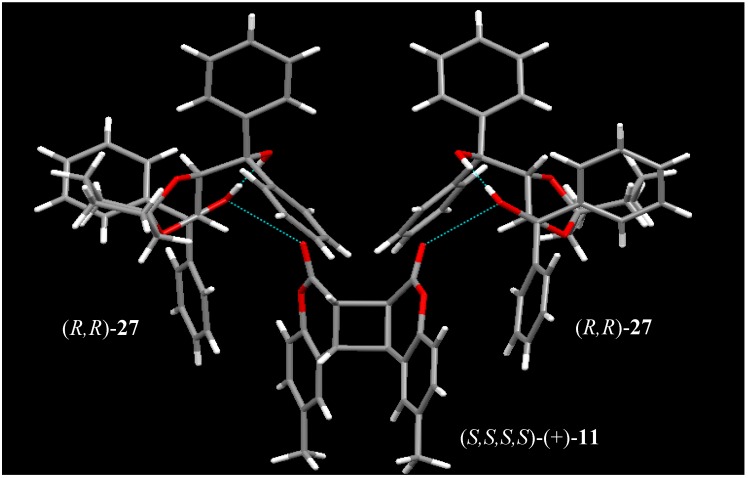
X-ray structure of the 2:1 complex of (−)-27 and the optically active *anti-head-head* dimer (+)-11.
